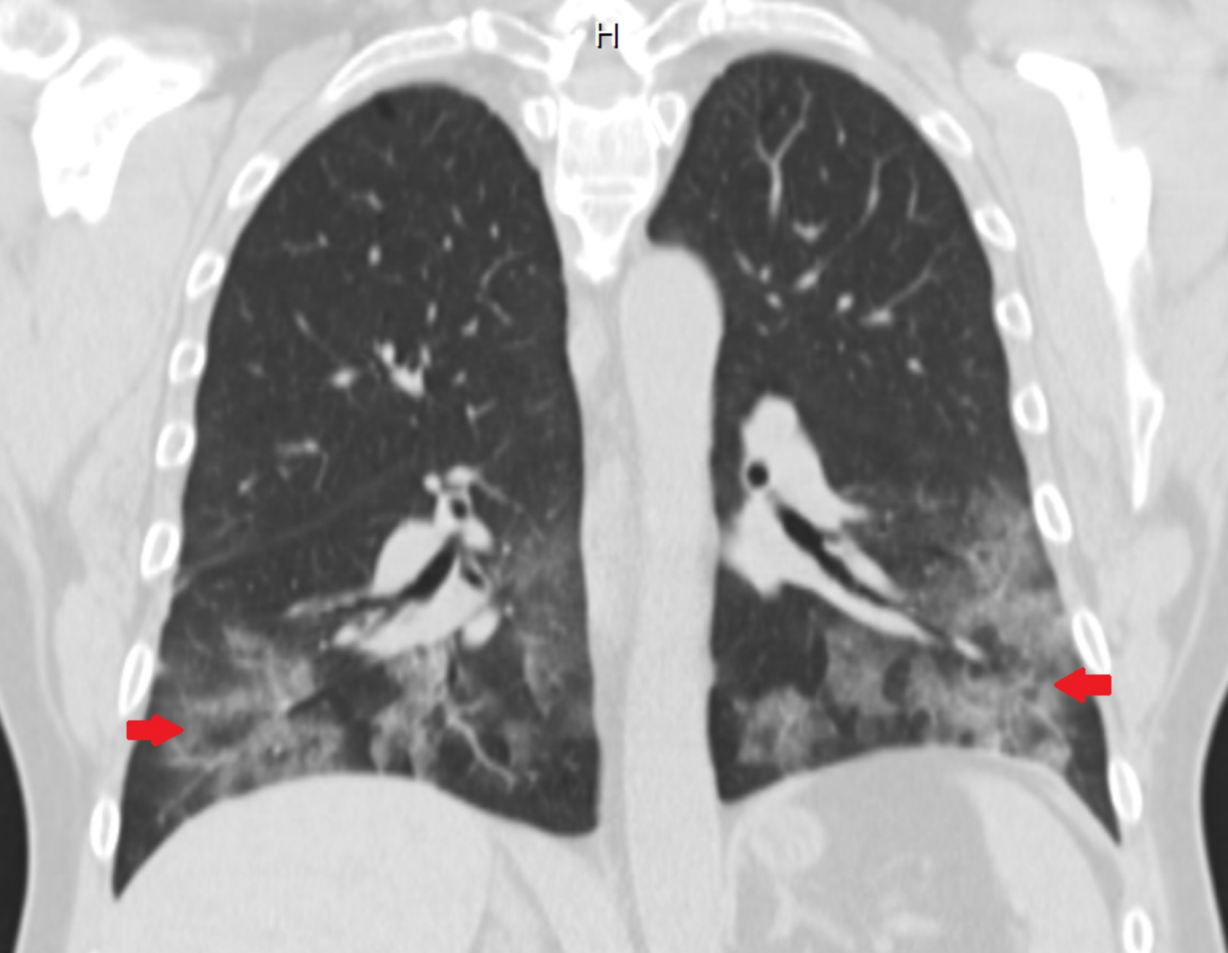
Coronal CT chest image showing bilateral basilar opacities (arrows)
